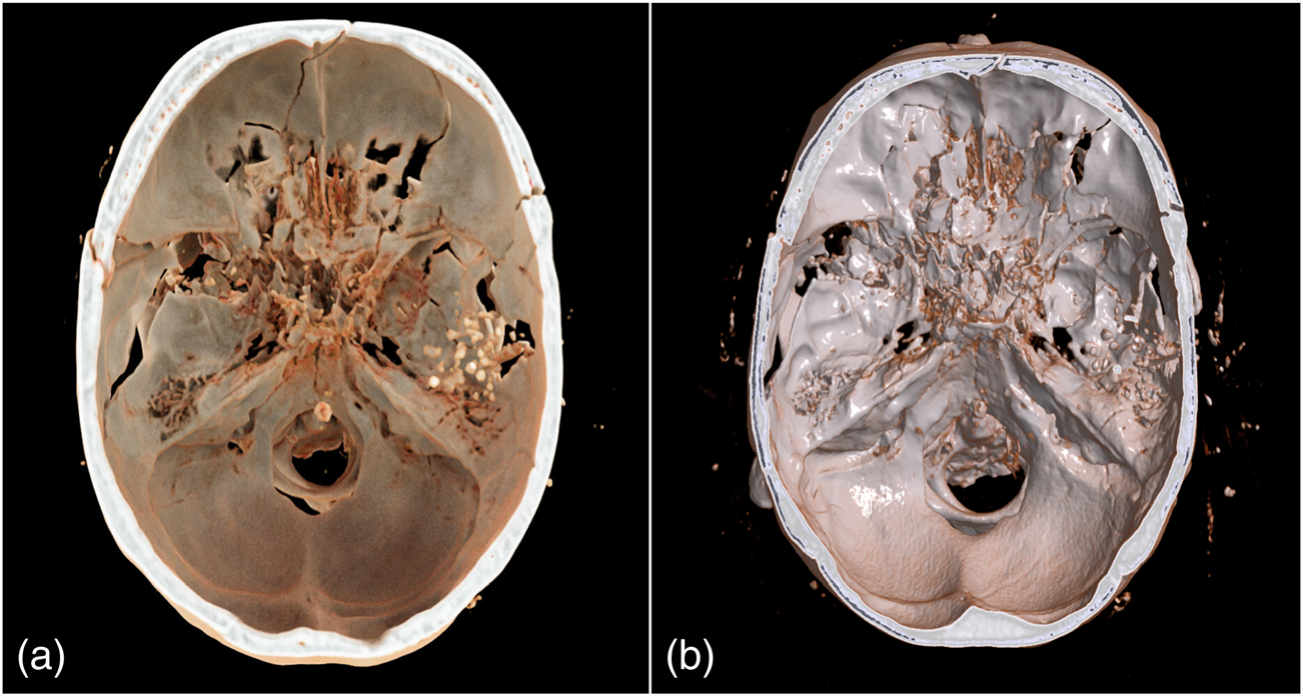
Non-enhanced 3-D CT images of a head shot injury. Both CR (a) and VR (b) show the bullets and extensive damage of the skull base. The more photo-realistic representation of anatomy with CR allows for an easy identification of the complex fracture course
